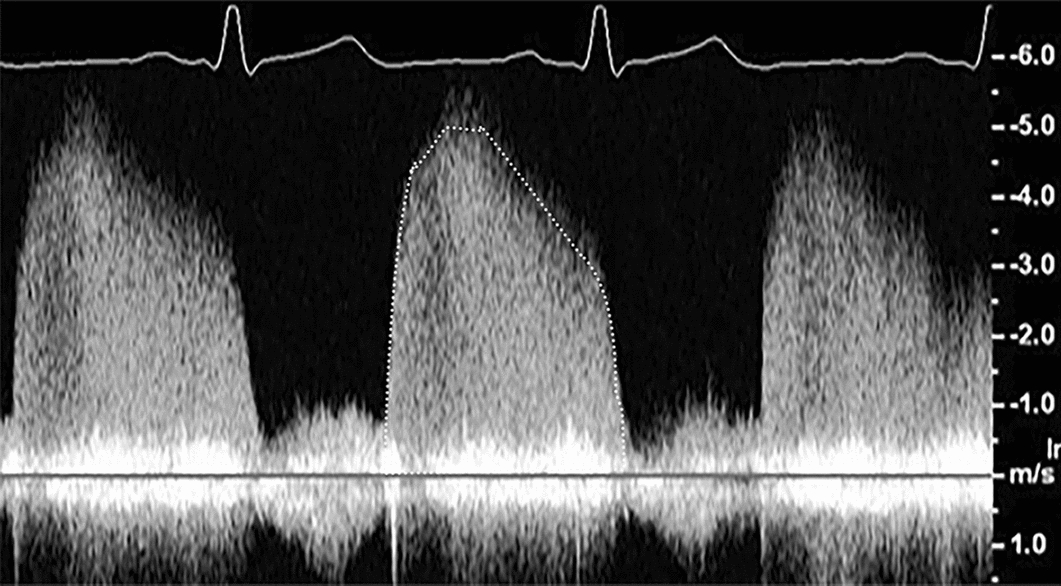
This image shows continuous wave Doppler of the aortic regurgitation trace. The velocity time integral (VTI) of the regurgitant waveform has been traced. The calculated VTI multiplied by the effective regurgitant orifice area (calculated as shown in Table 10) estimates the regurgitant volume. Of note, on some ultrasound systems, measurement of the VTI will also provide an estimate of the peak early diastolic velocity of the AR jet
